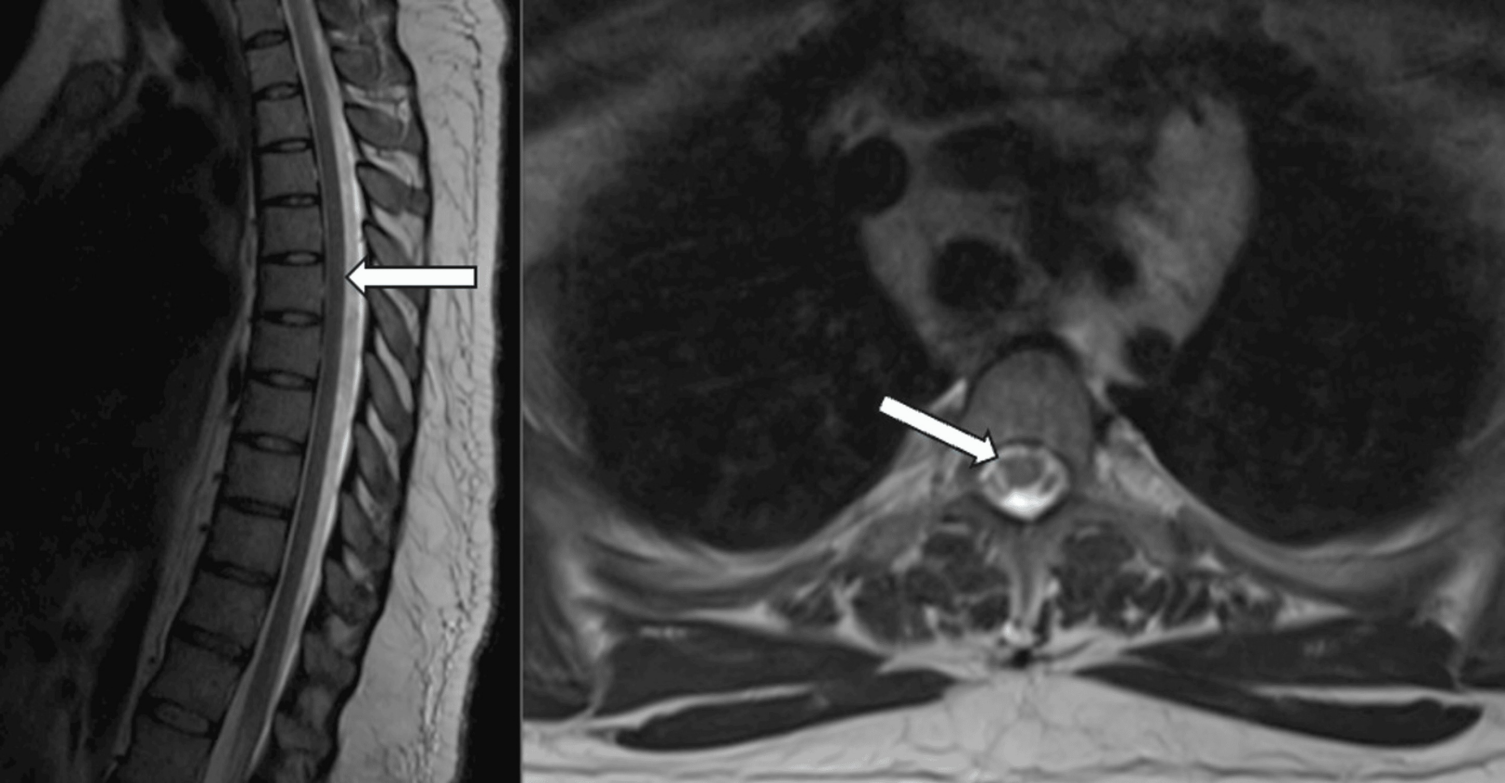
MRI of the thoracic spine sagittal view (left), axial view (right) showing multiple degenerative discs. No abnormal enhancements were observed.
